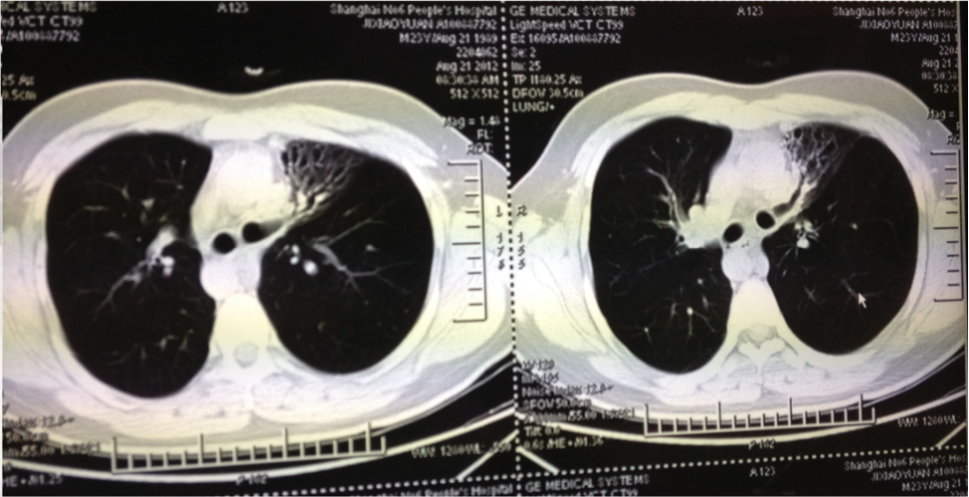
Chest computed tomography scan showing bronchiectasis in the local left lung
